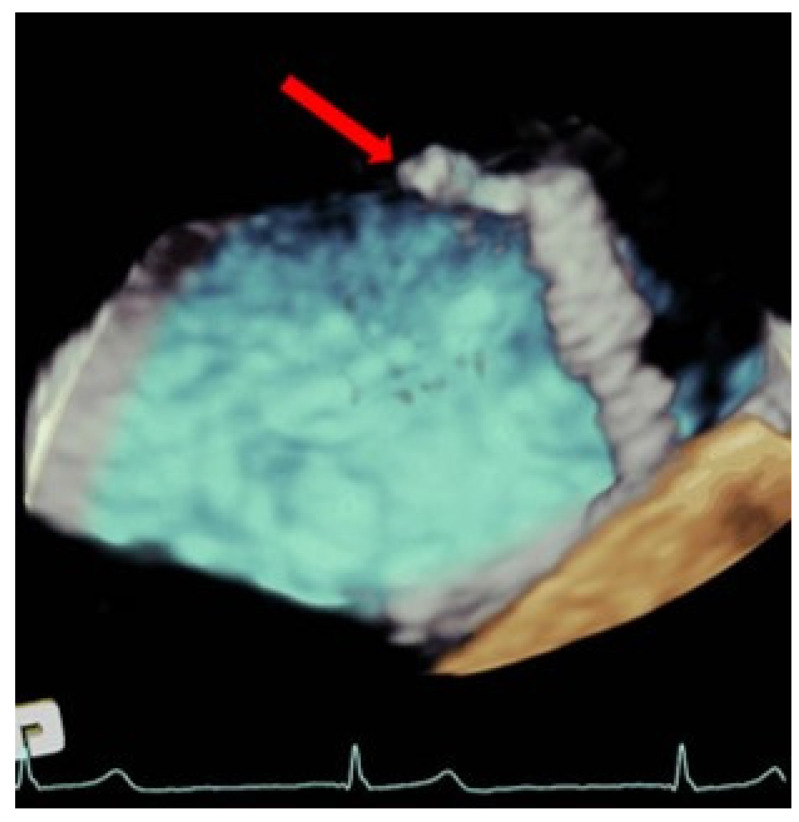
Three-dimensional transesophageal echocardiogram TEE image showing a large thrombus (red arrow) at the tip of the clip that is emerging from the delivery catheter within the left atrium.
